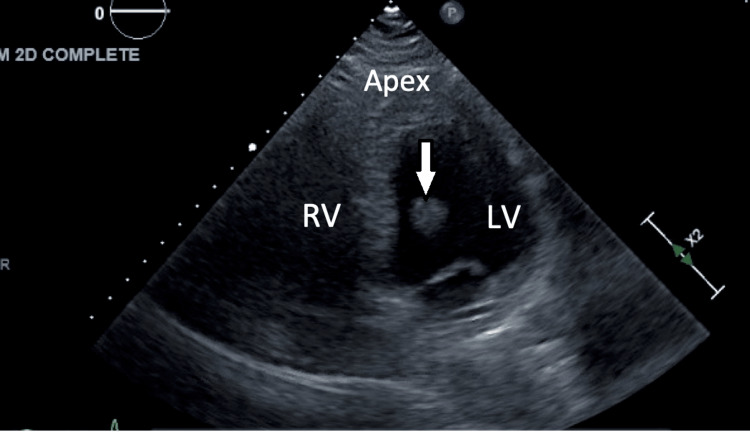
Transthoracic echocardiogram Two-chamber view on transthoracic echo revealing a mobile mass (white arrow) in the left ventricle (LV) RV: right ventricle
